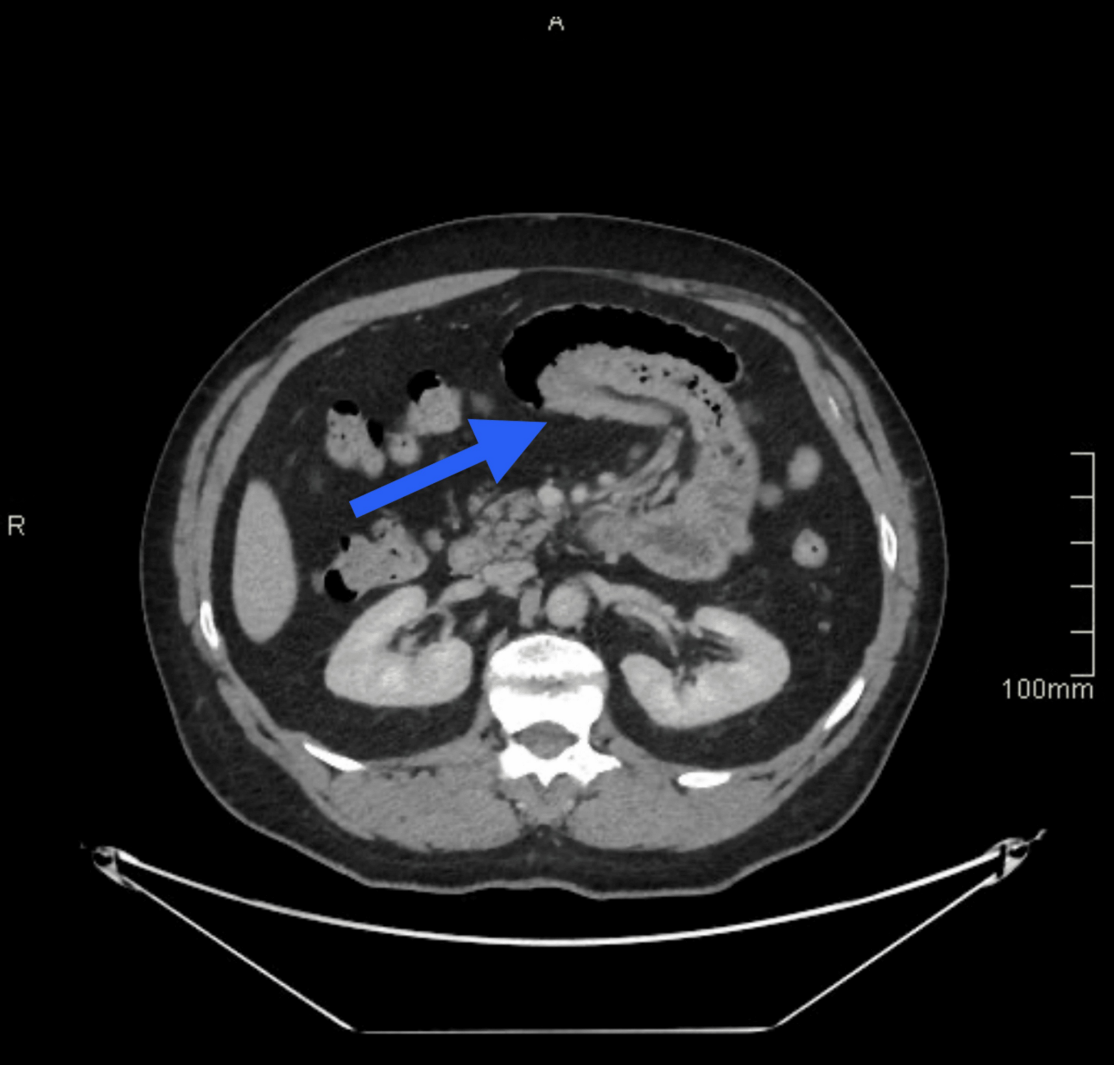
CT scan, axial view The arrow points to the intussuscepted segment.
